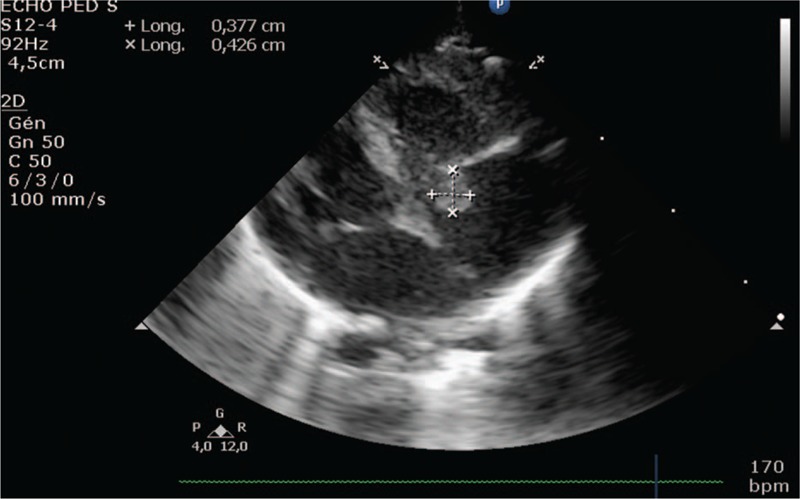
Vegetation on the tricuspid valve by echocardiography.
